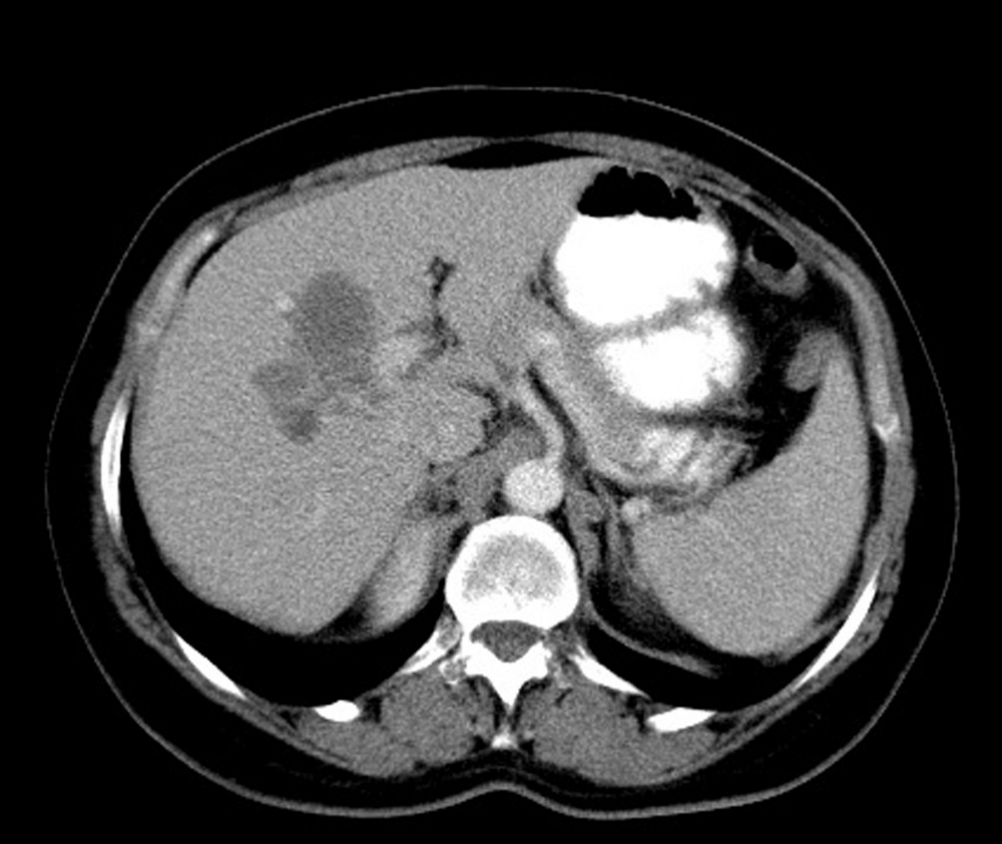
Post-treatment contrast-enhanced CT image in the portal phase 2 years later shows that the liver lesions had slightly regressed in size.
